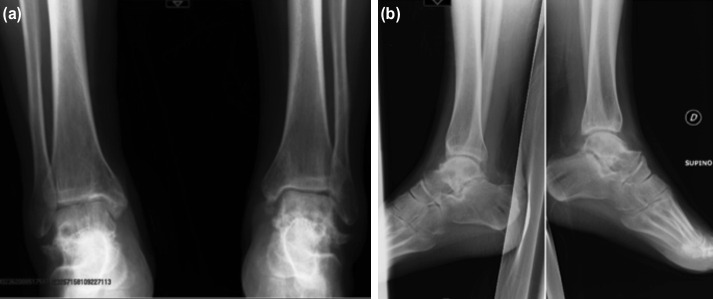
(a), (b) At 18 months of FU, radiograph after removal of the screws. Radiograph shows a severe bilateral talonavicular arthrosis and moderate arthrosis of the sub-talus joint. The patient was satisfied with his quality of life and he returned to fly with his aeroplane.
